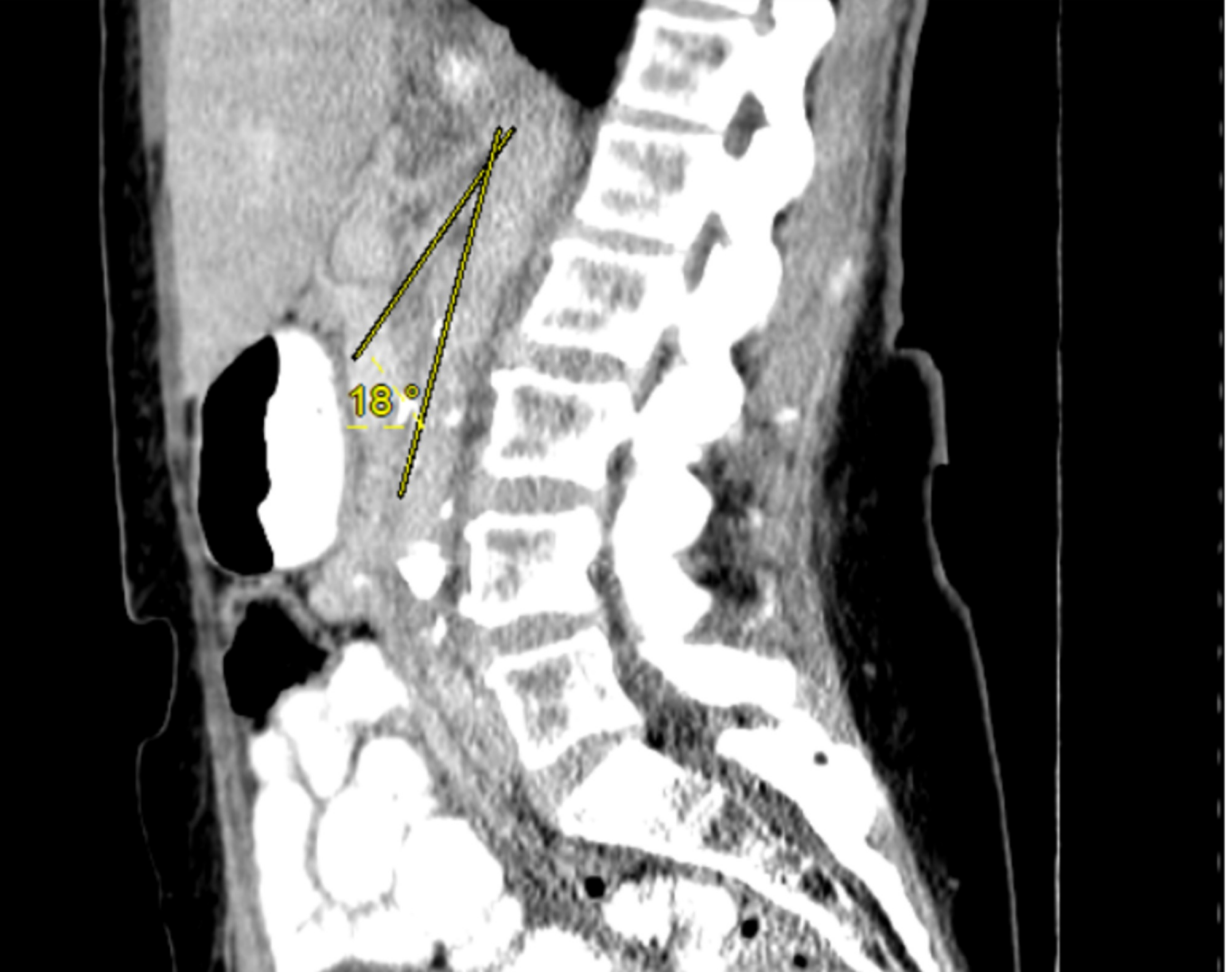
Sagittal CT scan of the abdomen/pelvis with oral and intravenous contrast. The angle between the SMA and the abdominal aorta is normally between 38° and 65°. In SMAS, the angle between the two vessels (i.e., aortomesenteric angle) is less than 25°. CT: computed tomography; SMA: superior mesenteric artery; SMAS: superior mesenteric artery syndrome.
